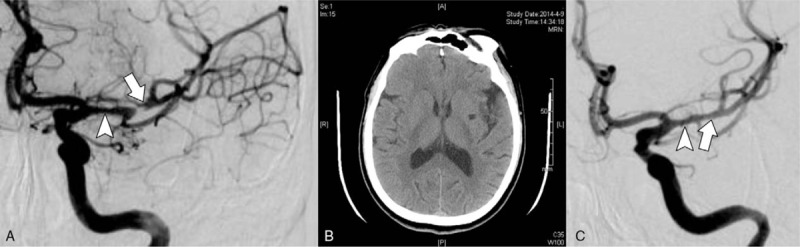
Brain digital subtraction angiography at admission revealed a remarkable stenosis (arrow) and a slight stenosis (arrowhead) in the left middle cerebral artery (A). Eleven days following anti-platelet treatment, the repeated brain CT showed hypodensity in the left putamen, suggestive of an old cerebral infarction (B). Repeated DSA (C) showed that the angiostenosis in the middle cerebral artery was significantly improved (arrow), and only slight stenosis remained (arrowhead).
